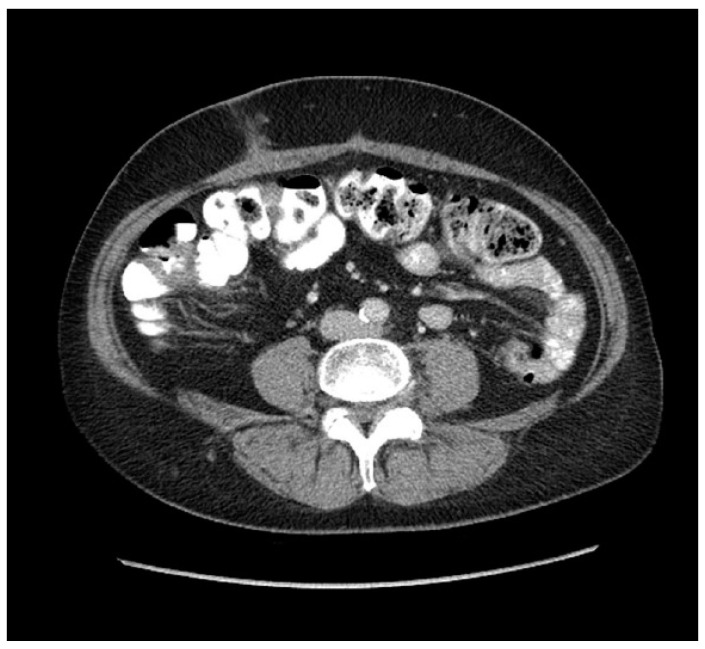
Surveillance computed tomography image after a prophylactic retrorectus synthetic mesh repair at the time of diverting ileostomy reversal.
